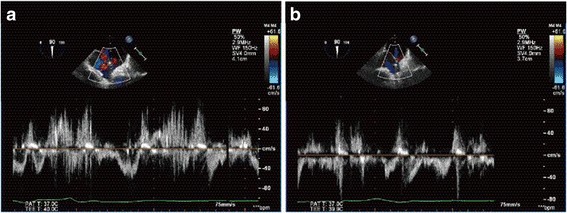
Pulse Doppler demonstrated increased right-to-left shunt in the right lateral decubitus position (a) compared with the left lateral decubitus position (b)
